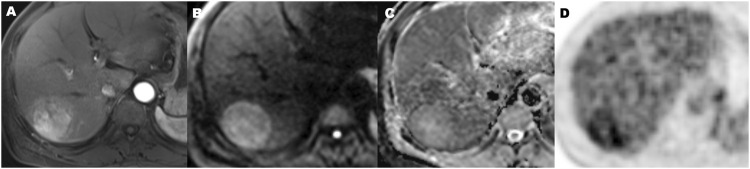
A 56-year-old hepatocellular carcinoma in liver segment VII man without microvascular invasion, demonstrating hypervascular enhancement pattern, low metabolic of SUVmax 3.5, MTV 72.2 cm^3^, TLG 196.5, TLR 1.9, and ADC value of 1.1 × 10^−3^ mm^2^/s. Contrast-enhanced MR in arterial phase image (A), diffusion-weighted MR image (B), ADC map (C), and ^18^F-FDG PET (D) (MTV, metabolic tumor volume; TLG, total lesion glycolysis; TLR, ratio of tumor-to-liver SUV; ADC, apparent diffusion coefficient).
